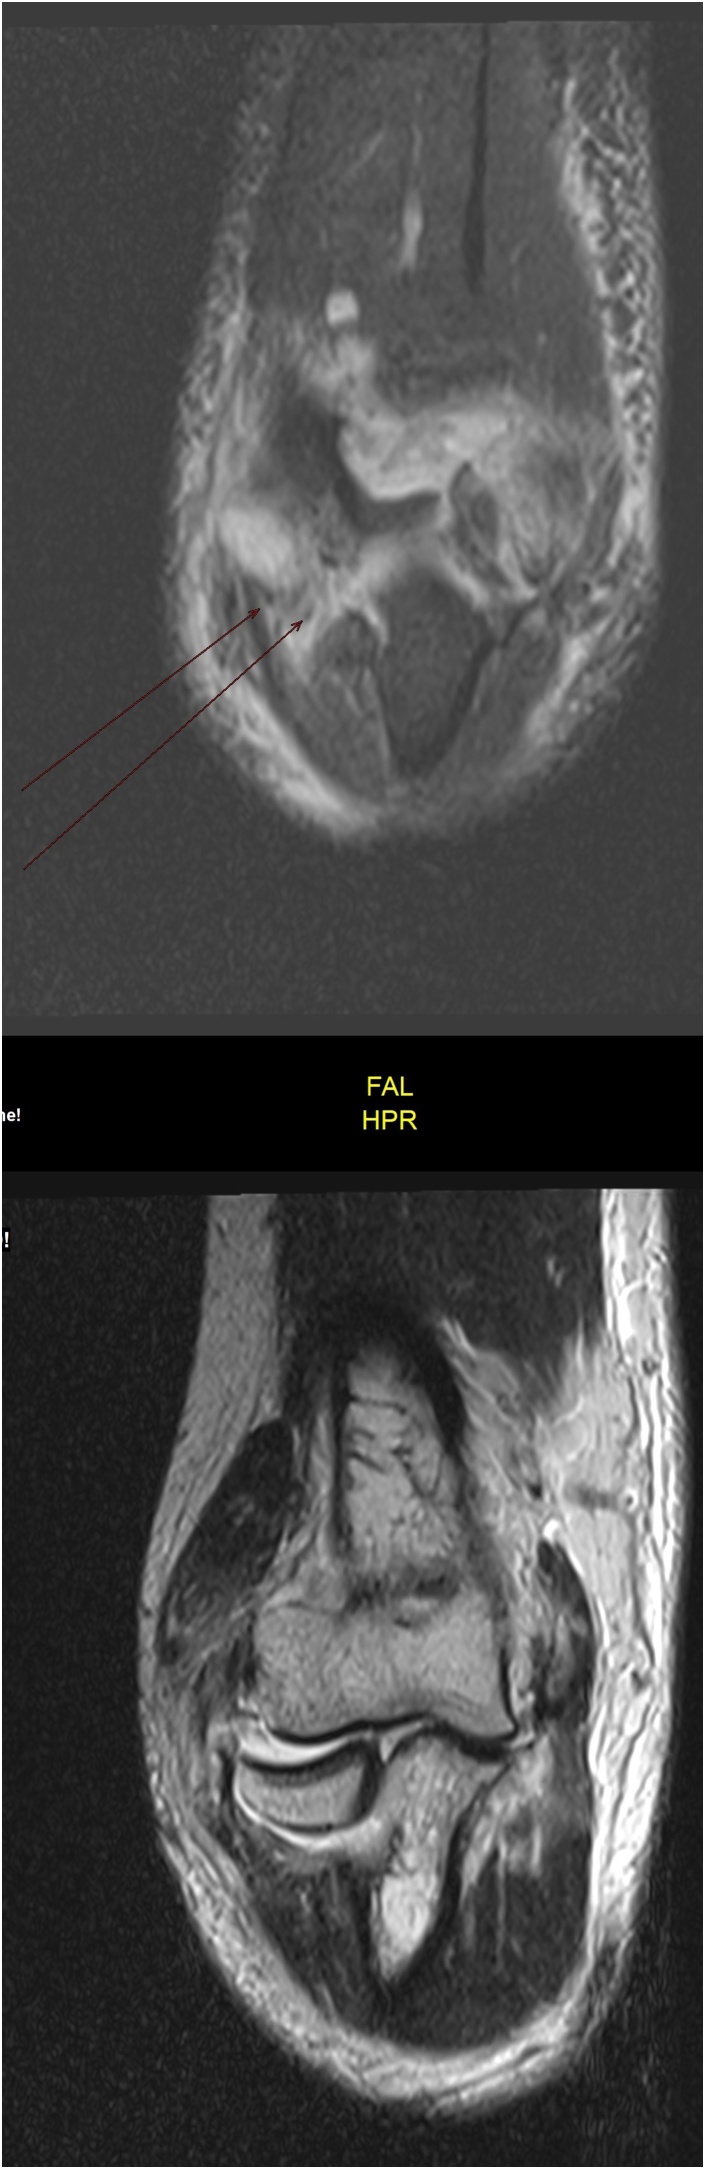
Left elbow T1, T2 weighted MRI showed a coronoid fracture, medial epicondylar fracture, and radial and ulnar collateral ligaments tear.
